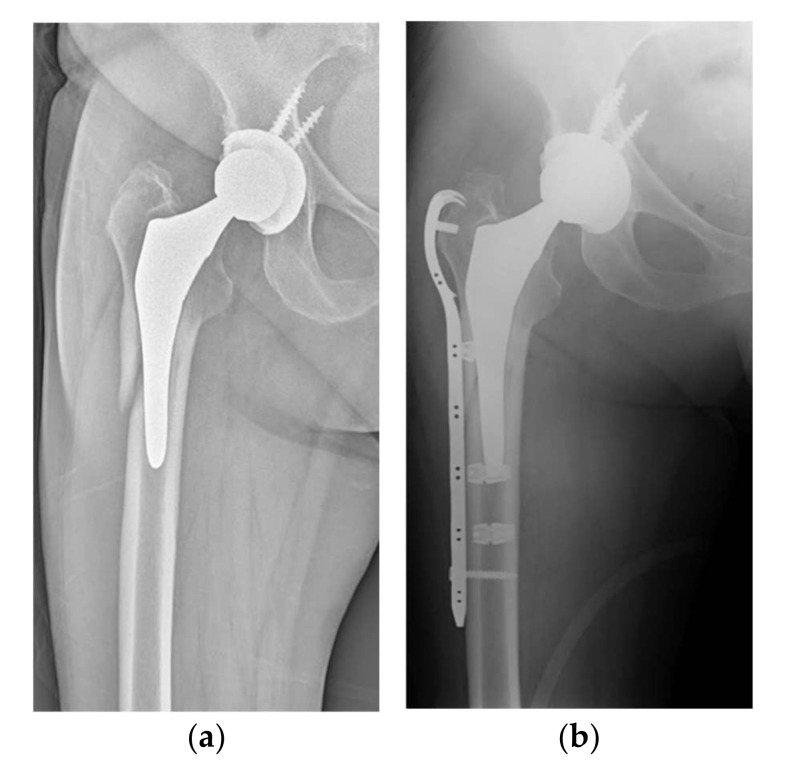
(a) Preoperative X-ray of type B1 fracture in 76-year-old patient; (b) postoperative 3-month follow-up after placement of a plate with UHMWPe cables.
